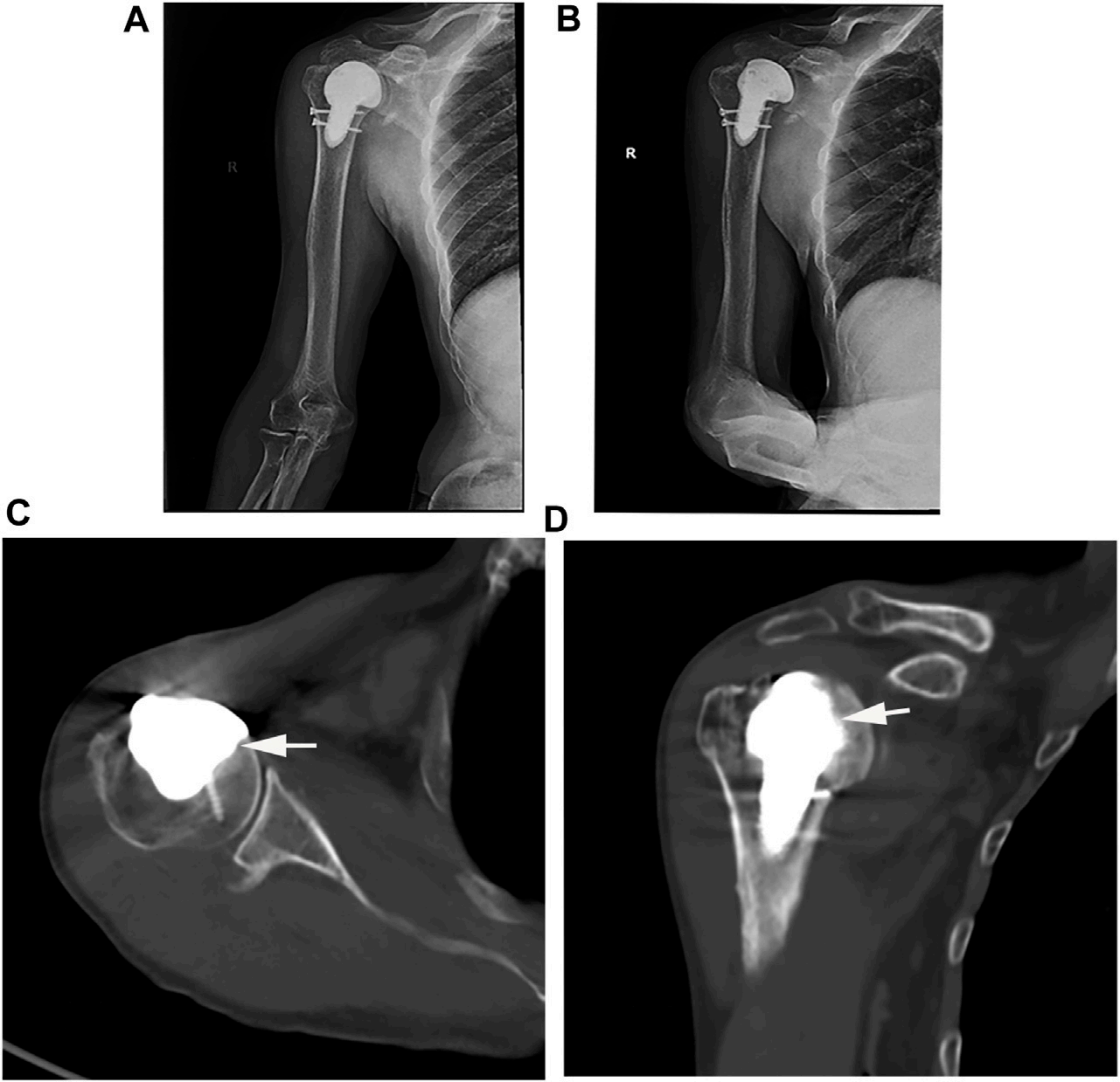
(A, B) Postoperative X-ray and (C, D) CT examination (White arrow: successful osseointegration).
